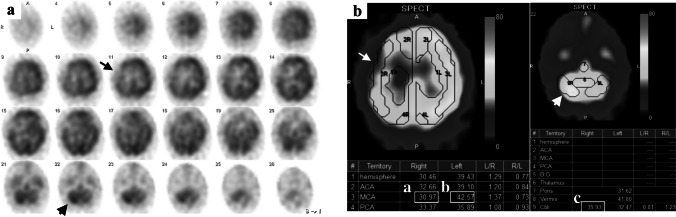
Single-photon emission computerized tomography of the same patient in Fig. 1. a, b SPECT reveals decreased rCBF in the right MCA territory (arrow) and the rCBF in the right cerebellum (arrowhead). b The CBF analysis software reveals an AI% of 73% (i.e., 30.97 (a) divided by 42.57 (b)) and an rCBF% of 78.7% (i.e., 30.97 (a) divided by 39.93 (c)). AI%, asymmetric index; CBF, cerebral blood flow; MCA, middle cerebral artery; rCBF, regional cerebral blood flow; SPECT, single-photon emission computerized tomography
